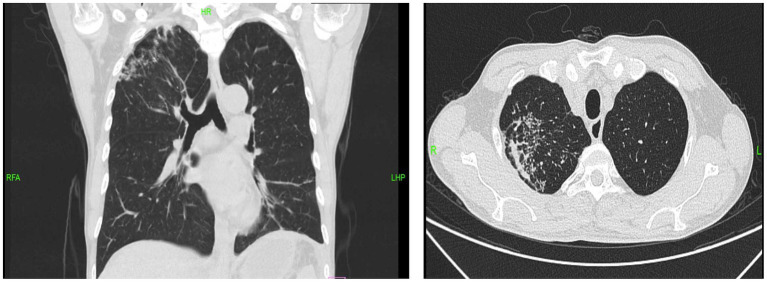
Chest CT shows multiple patchy and nodular opacities in the right upper and left lower lobes, with partial calcification and a tree-in-bud pattern adjacent to pleural thickening.
